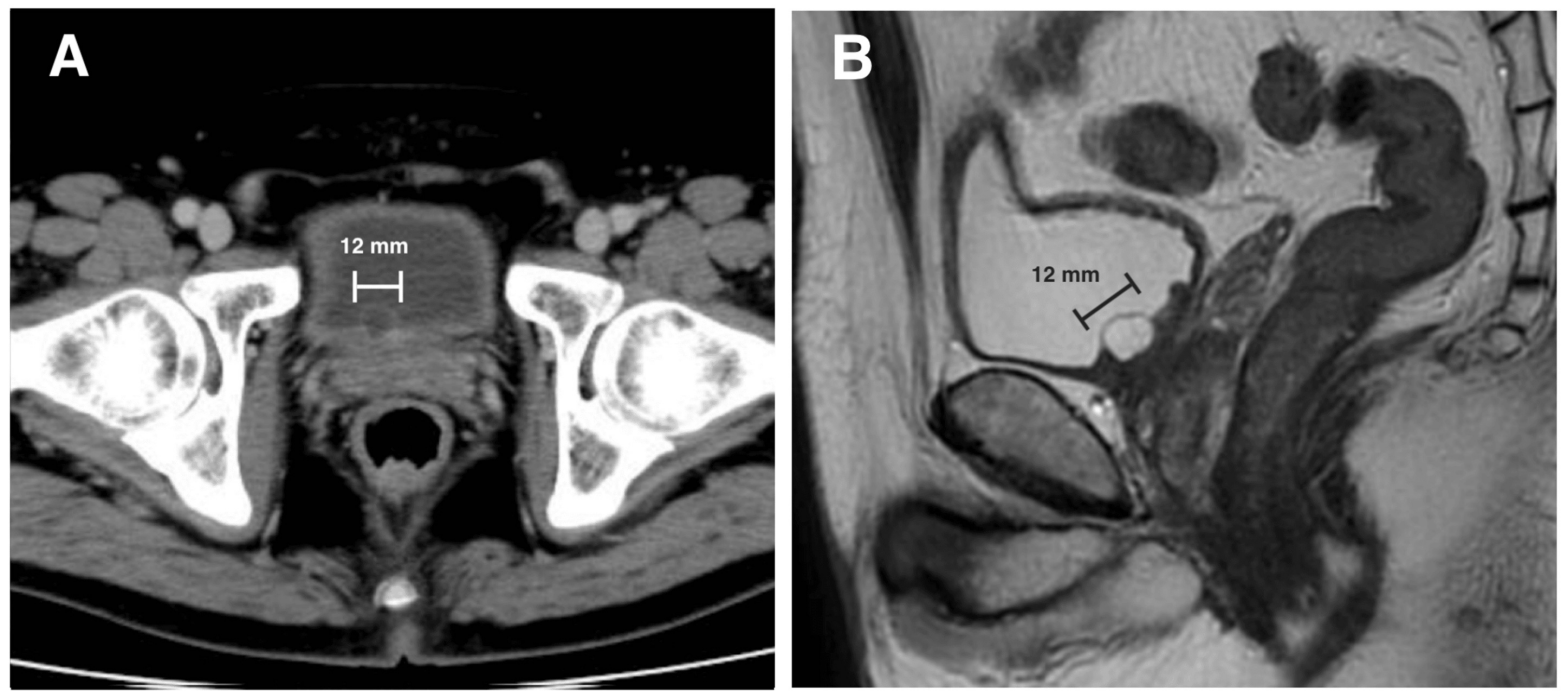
Axial contrast-enhanced computed tomography image (A) and sagittal magnetic resonance imaging T2-weighted image (B) of the bladder.
